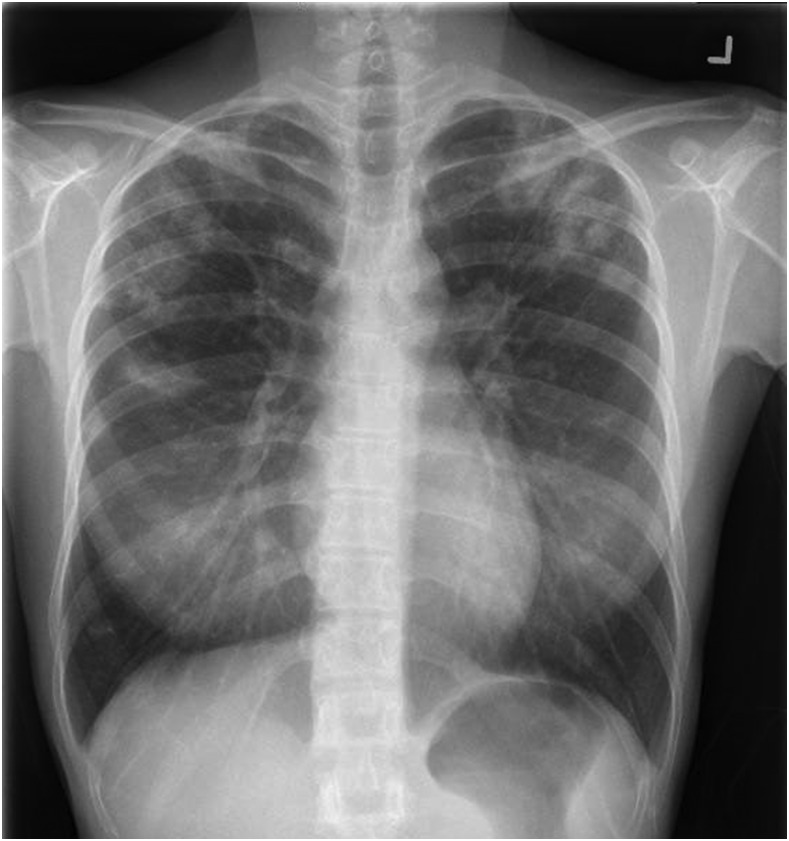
Chest radiograph shows nodular opacities over the upper and mid-zones with cavitation in the left upper zone.
